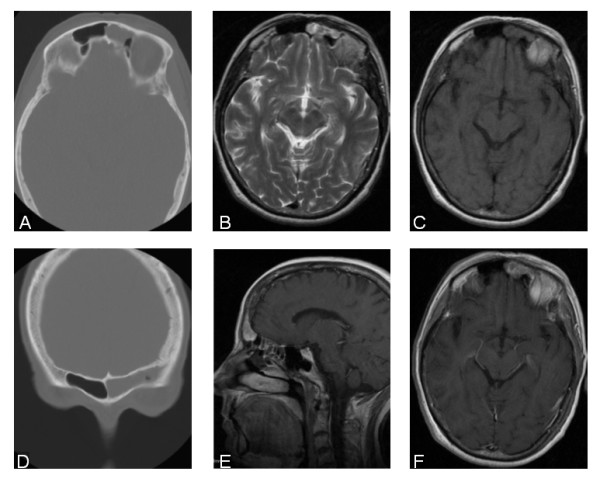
Sinonasal meningeoma. CT scan in bone window (A, D) reveals a soft tissue mass in the left frontal sinus without any signs of bone destruction or reaction. Axial T2 weighted images (B), T1 weighted images before (C) and after contrast administration (E, F) show a contrast enhancing soft tissue mass being slightly inhomogeneous in the left frontal sinus without any intracranial meningeal enhancement.
